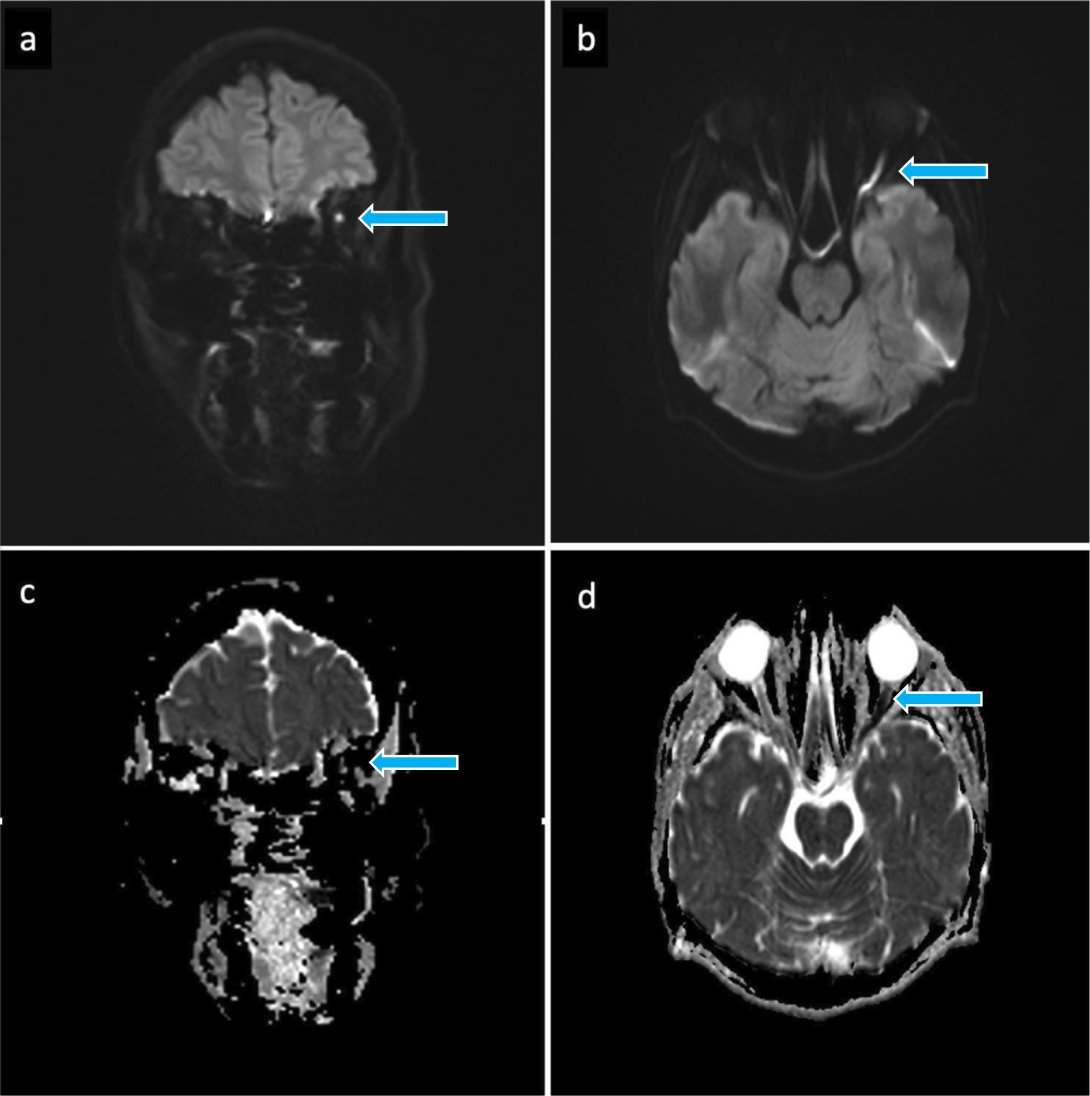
MRI of the brain two months after the HA filler injection. Coronal (a) and axial (b) diffusion weighted imaging (DWI) b = 1000 images and corresponding apparent diffusion coefficient (ADC) maps (c, d) corresponding to stable MRI findings
